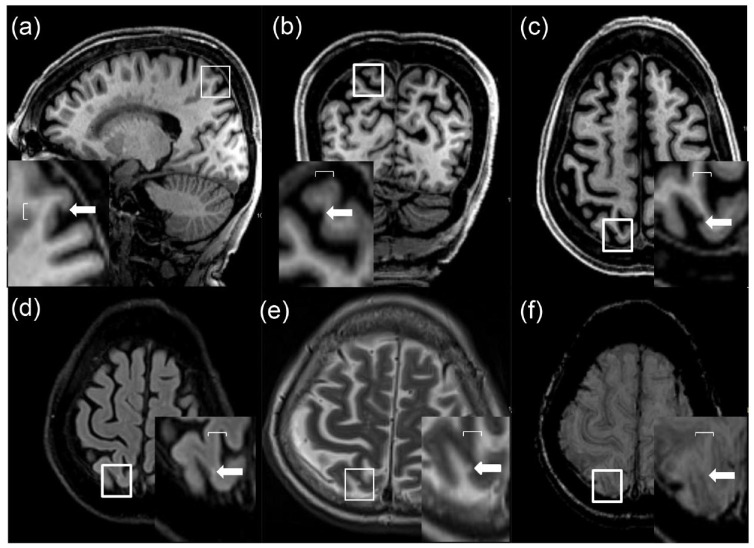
Cortical cerebral microinfarcts on 3T MRI. They are defined as hypointense
